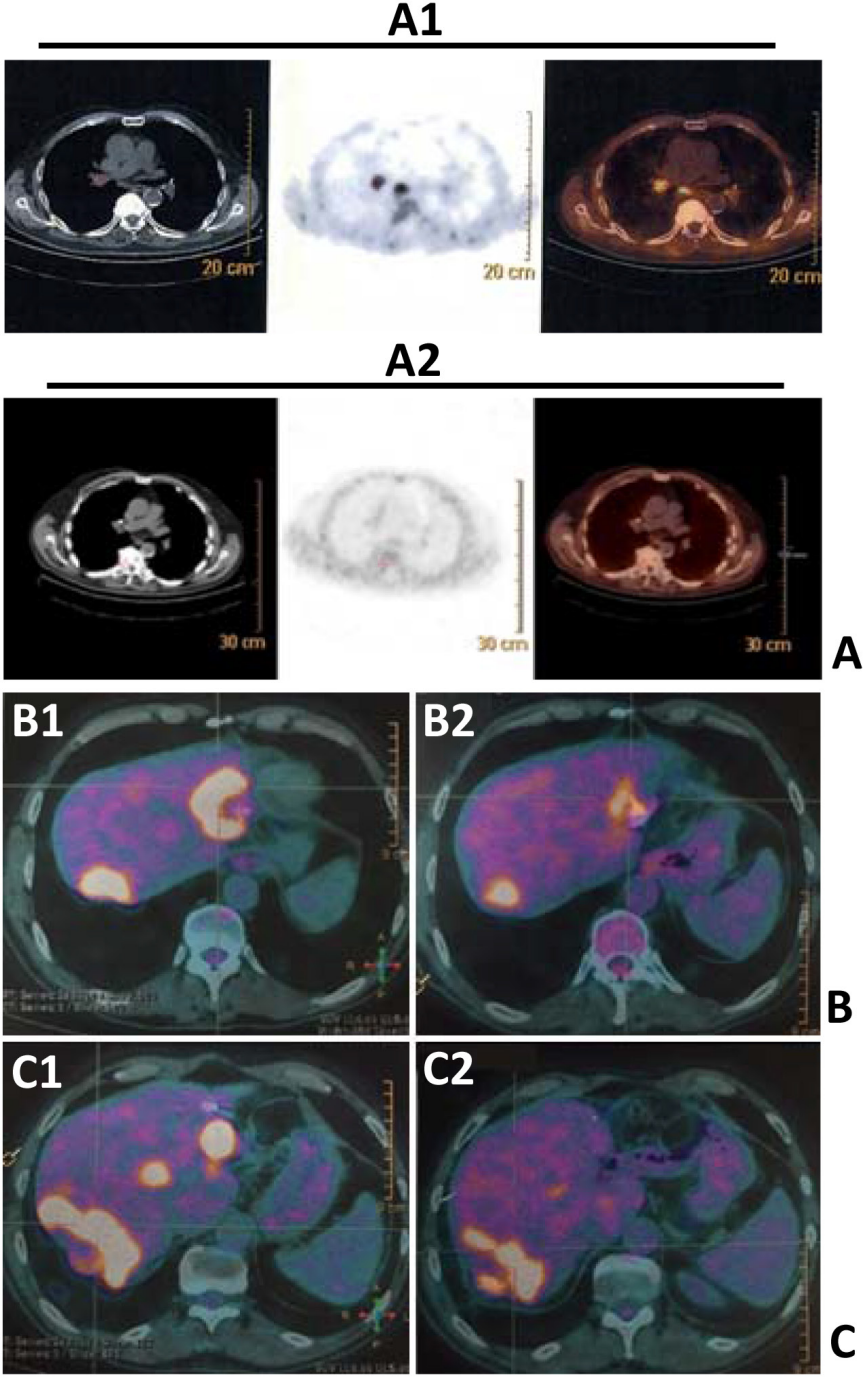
FDG-PET scans showing FDG uptakes before (A1, B1 and C1) and after SRT (A2, B2 and C2) in lungs (A1 vs A2) and liver metastases (B1 vs B2; C1 vs C2) of three patients (panels A, B, C).
